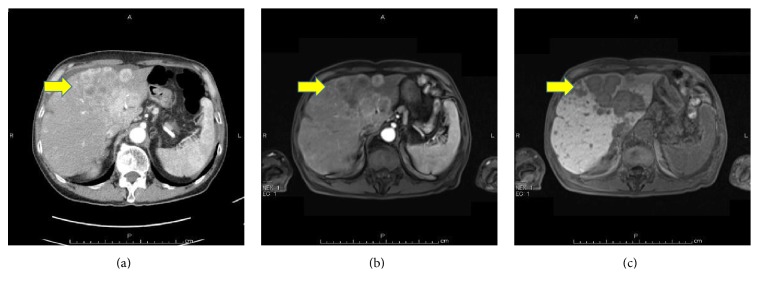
Dynamic contrast-enhanced computed tomography (CT) scans showed multiple masses in the patient's liver, suggesting intrahepatic cholangiocarcinoma (ICC; (a), arrow). His liver tumors showed an enhancement of the peripheral zone in the early phase ((b), arrow) and a low intake in the hepatobiliary phase ((c), arrow) in a gadolinium ethoxybenzyl diethylenetriamine pentaacetic acid- (Gd-EOB-DTPA-) enhanced magnetic resonance imaging (MRI).
